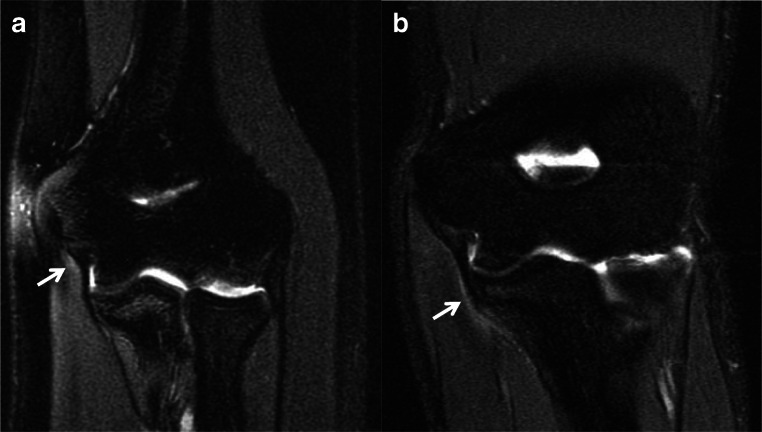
Coronal T2 MR images of the elbow in different patients demonstrate periligamentous edema about the proximal (a, arrow) and distal (b, arrow) UCL consistent with low-grade sprains
